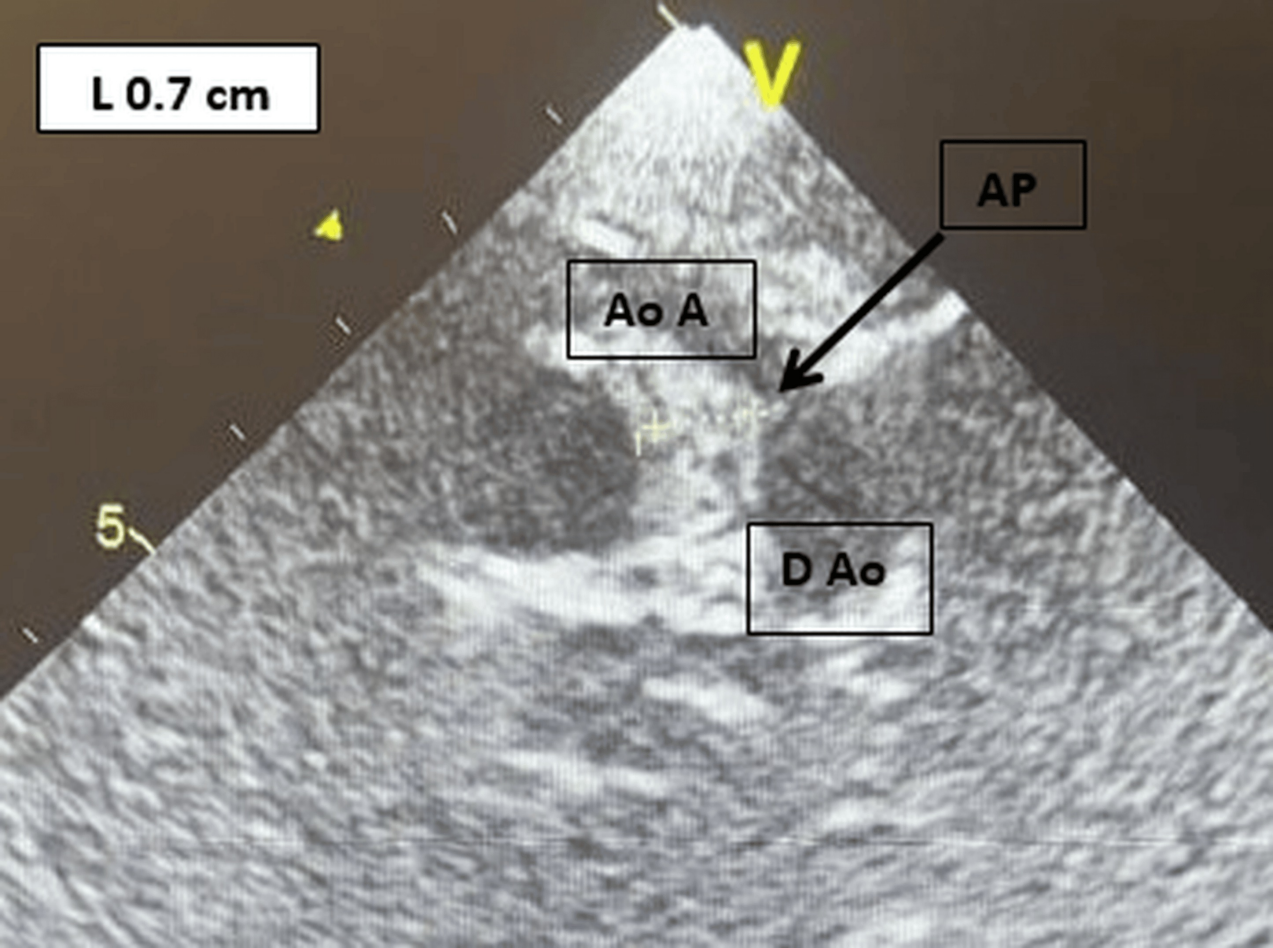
Two-dimensional echocardiographic image from the suprasternal window of large atherosclerosis plaque in the aortic arch. The plaque is 7 mm thick. Ao A is the aortic arch, AP is the atherosclerosis plaque, and D Ao is the descending aorta. The arrow indicates the atherosclerotic plaque in the aortic arch.
